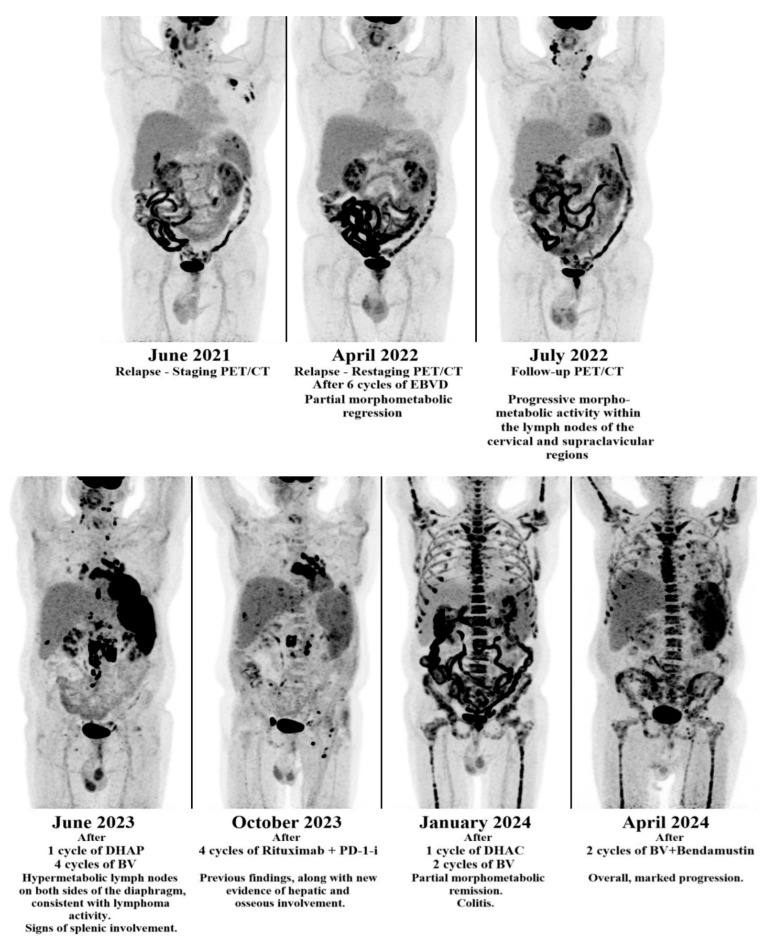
Positron emission tomography–computed tomography (PET/CT) scan results of the second patient with common variable immunodeficiency (CVID)-associated Hodgkin lymphoma (HL). The images demonstrate initial partial responses followed by disease progression despite multiple lines of therapy, including brentuximab vedotin (BV), programmed cell death protein 1 (PD-1) inhibitor, rituximab and chemotherapy. DHAP: dexamethasone, high-dose cytarabine and cisplatin; BV: brentuximab vedotin; PD-1-i: programmed cell death protein 1 inhibitor; DHAC: dexamethasone, cytarabine, carboplatin.
